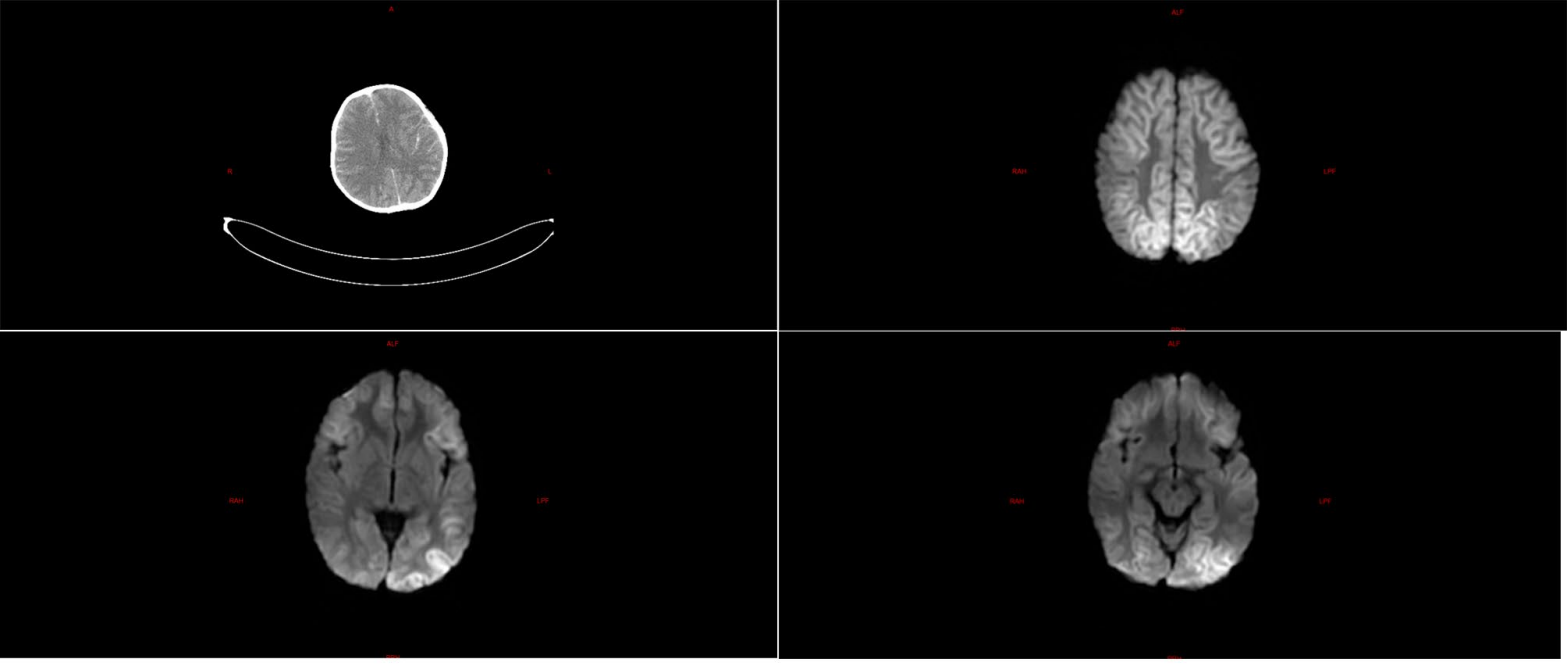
a: Enhanced brain CT image of case 2, showing diffuse swelling of bilateral cerebral hemispheres, shallower sulci, decreased cortical density, and linear enhancement in bilateral temporal lobe sulci; b, c, and d are brain MR DWI sequences of case 2, showing symmetrical diffuse hyperintensities in both cerebral hemispheres, mainly involving the cortex. The patient experienced a rapid deterioration of condition shortly after admission, manifesting cortical stiffness within a short timeframe and developing central diabetes insipidus. Unfortunately, the patient succumbed to multiple organ failure in the end
